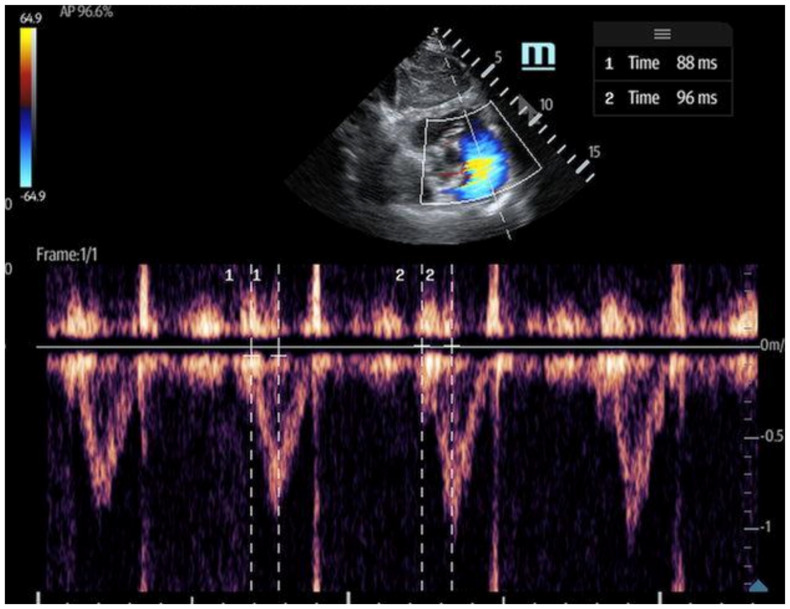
Pulmonary acceleration time (PAT) measured in the subcoastal short axis, and PW doppler activation measured as the interval between the onset of pulmonary flow and peak velocity.
